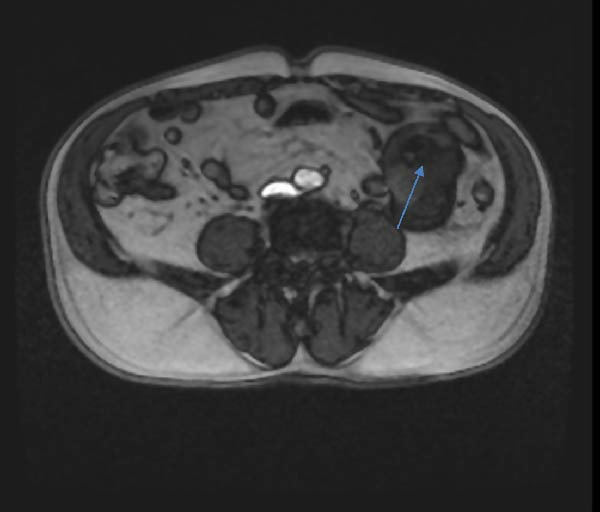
MRI of the small bowel showing a lesion that is mainly T1 hyperintense and T2 hypointense. An irregular segmental wall thickening is noted without proximal dilatation above the stenosis.
